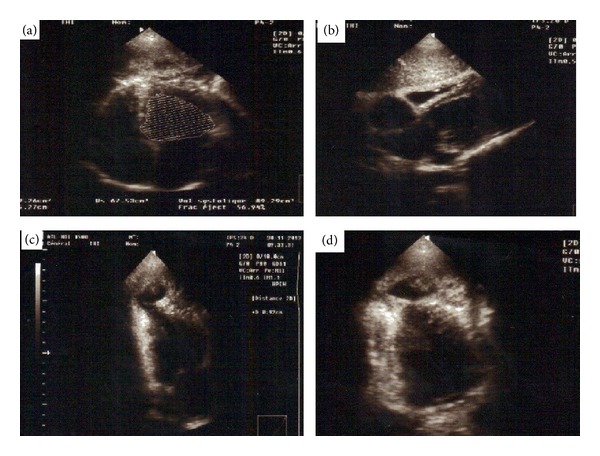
Cardiac ultrasound findings. (a) Calculation of ejection fraction from 57% in Simpson 4 cavities. (b) Cutting subcostal objectifying the left ventricle and the pseudoaneurysm with no notion of communication. ((c) and (d)) Apical view: collar diameter was 9 mm, and presence of a heterogeneous structure suggestive of thrombus lining the pseudoaneurysm was noted.
